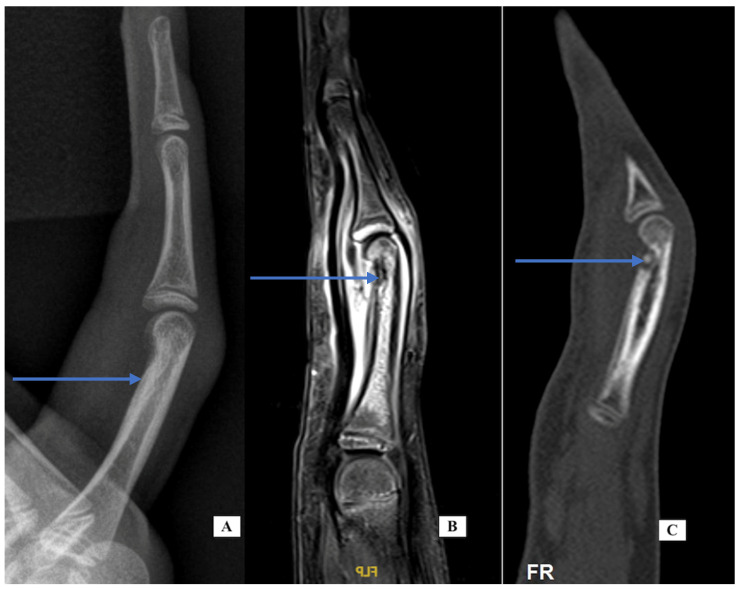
Case 4—X-ray lateral of the finger (A) demonstrating cortical thickening; MRI sagittal of the finger (B) demonstrating bone marrow edema distal P1, irregularity of the distal cortex (blue arrow) and generalized soft tissue swelling; CT sagittal of the finger (C) demonstrating lucency with a central calcification consistent with nidus.
